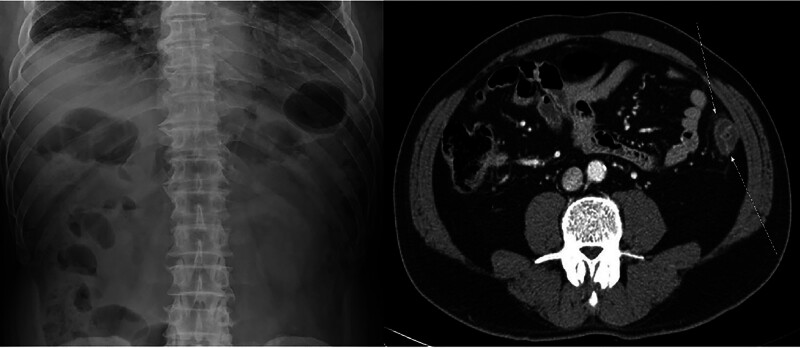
(Left) Plain abdominal radiograph without free air. (Right) Contrast-enhanced computed tomography demonstrating focal circumferential wall thickening of the descending colon (25 mm) without pneumoperitoneum, consistent with post-polypectomy electrocoagulation syndrome.
